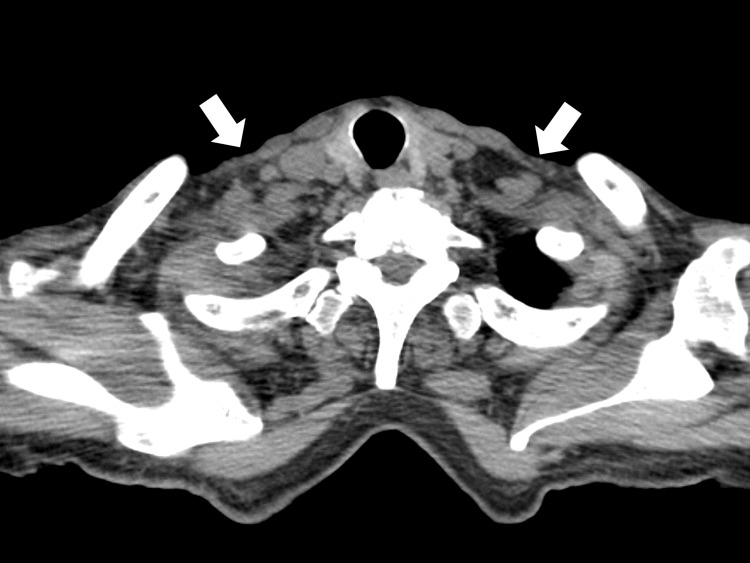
Computed tomography image of the chest showing lesions of subclavian lymphadenopathy (white arrows).
